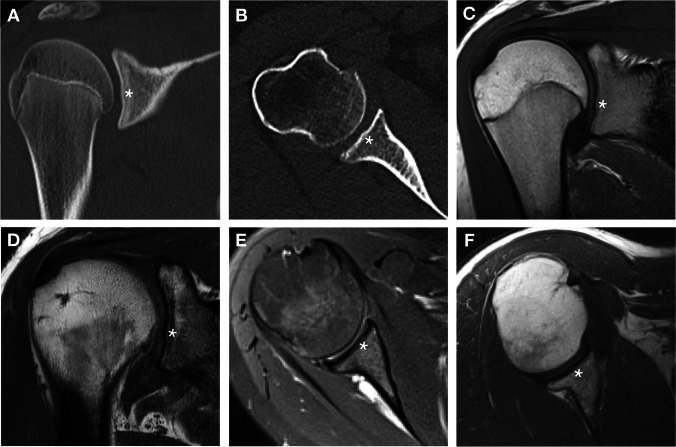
The appearance of the tubercle of Assaky (asterisk) on CT and MRI scans. CTs showing coronal slice of a 15-year-old male (A) and axial slice of a 69-year-old female (B). MRIs showing coronal slices of a 17-year-old female (C) and 31-year-old male (D); and axial slices of a 30-year-old female (E) and 52-year-old male (F)
